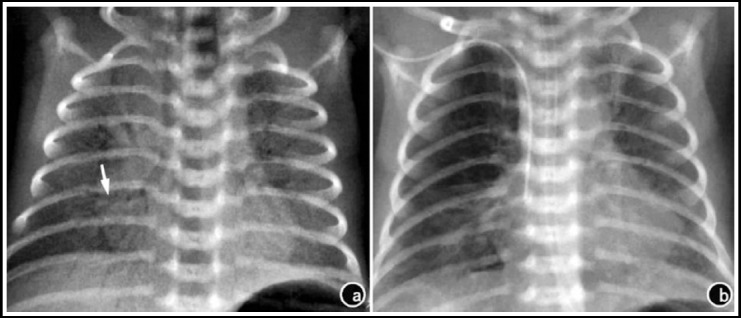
Pulmonary X-ray results of neonate No. 1 before and after treatment. a) 3 h after birth, before treatment; b) 30 h after PS treatment.
